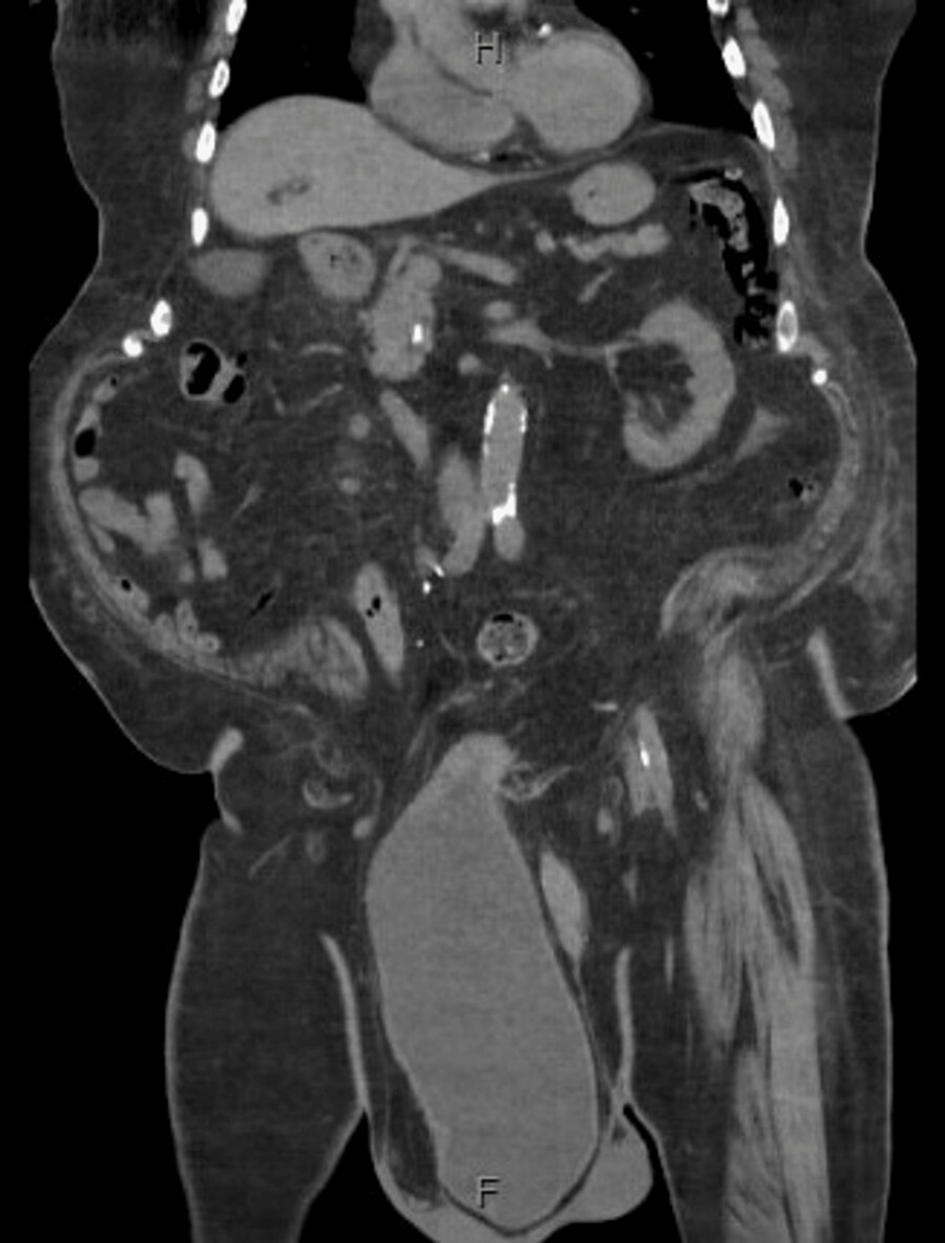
CT scan of abdomen and pelvis coronal view showing a large right inguinal hernia containing most of the bladder and the appendix CT: Computed tomography
